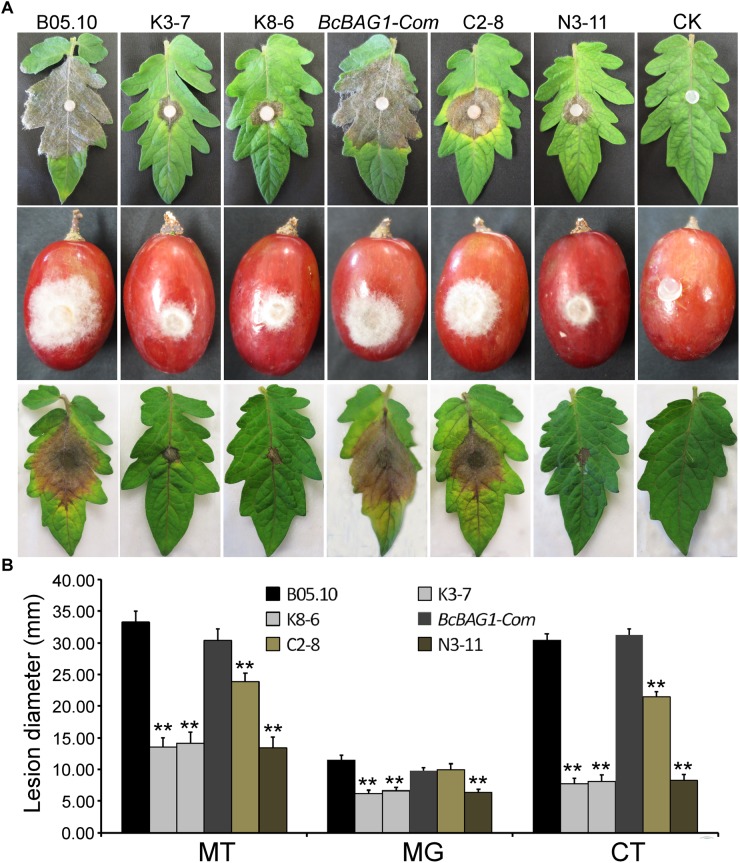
BcBAG1* deletion mutants attenuated pathogenicity. (A) Inoculation assays were implemented on different host plants. Mycelial plugs from PDA after 3 days growth were inoculated on detached tomato leaves (Moneymaker) (upper panel) and wounded grapes (middle panel). The disease phenotype was recorded 4 days post inoculation. Conidial suspensions (1.0–1.5 × 10^5^ spores/ml) were inoculated on detached tomato leaves (Moneymaker) (lower panel) and the disease symptom was recorded 7 days post inoculation. (B) Quantification of lesion diameters of above inoculation. MT, mycelial plugs were inoculated on tomato leaves; MG, mycelial plugs were inoculated on grapes; CT, conidial suspensions were inoculated on tomato leaves. Wild-type strain (B05.10), Δ*Bcbag1* mutant lines (K3-7 and K8-6), and complemented strains (*BcBAG1-Com*, C2-8 and N3-11); CK, negative control. Error bars represent the standard deviations from three independent experiments and asterisks indicate statistical significance (*P* < 0.01).
